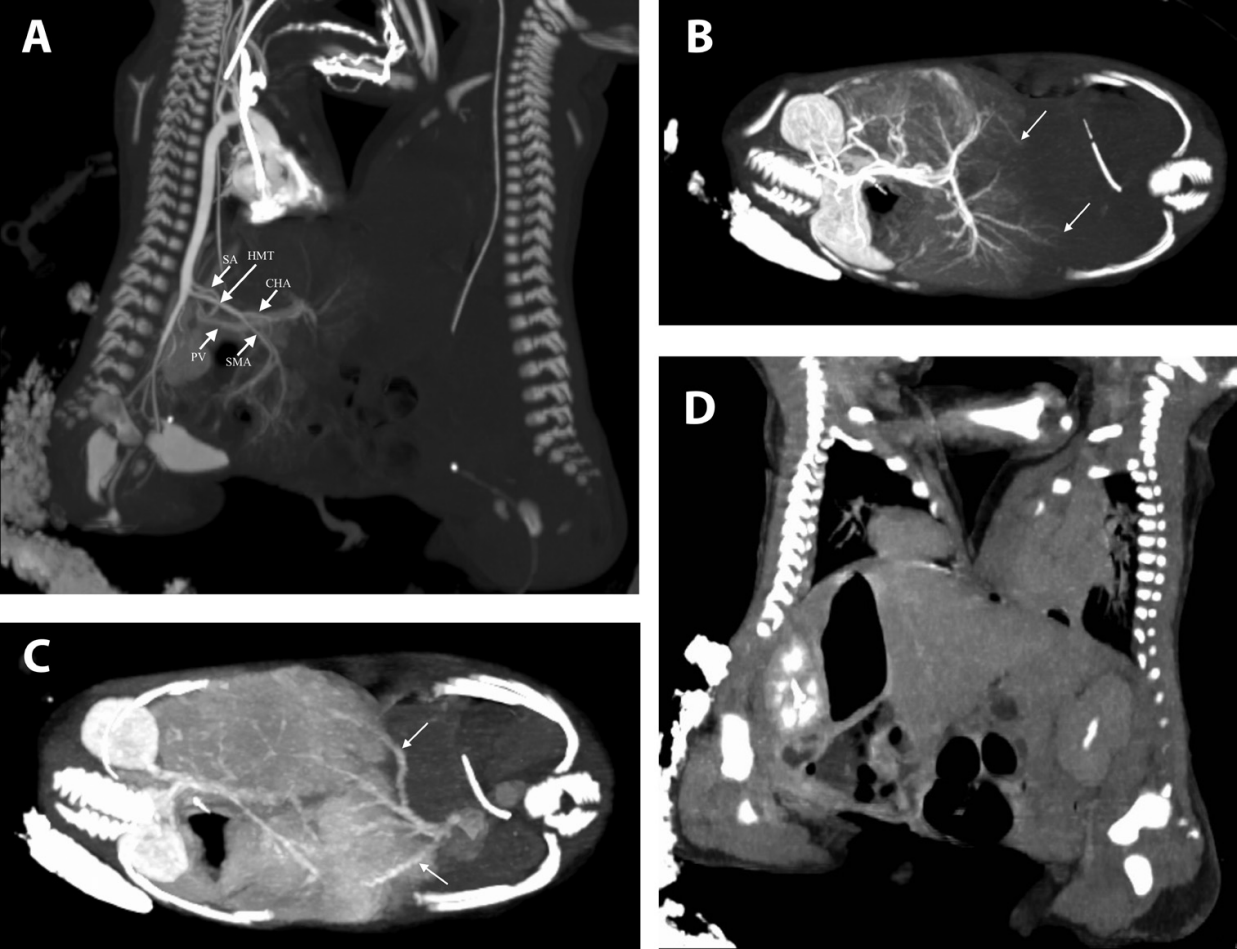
Dynamic contrast-enhanced computed tomography with multi-projection volume reconstruction images. (A) A sagittal image of the arterial phase showing the separate origin of the splenic artery (SA), a common origin of common hepatic artery (CHA), and the superior mesenteric artery (SMA) representing the hepato-mesenteric trunk (HMT), and normal anatomy of twin A's portal vein (PV). (B) An axial image of the arterial phase showing enhanced hepatic vein branches in twin B (arrows) communicating with twin A's portal branches, representing portosystemic crossover shunts. (C) A portosystemic crossover shunt is confirmed by pronounced opacification of hepatic veins in twin B (arrows) on an axial image of the venous phase. (D) A delayed image showing persistent nephrogram in twin A and normal pyelographic phase with a contrast in the renal pelvis in twin B.
